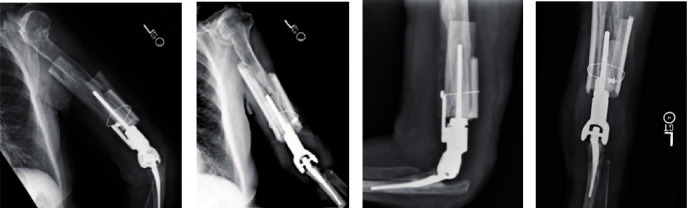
(a–d) 12-month postoperative X-ray images: (a) lateral humerus X-ray; (b) AP humeral X-ray; (c) lateral elbow X-ray; (d) AP elbow X-ray.
